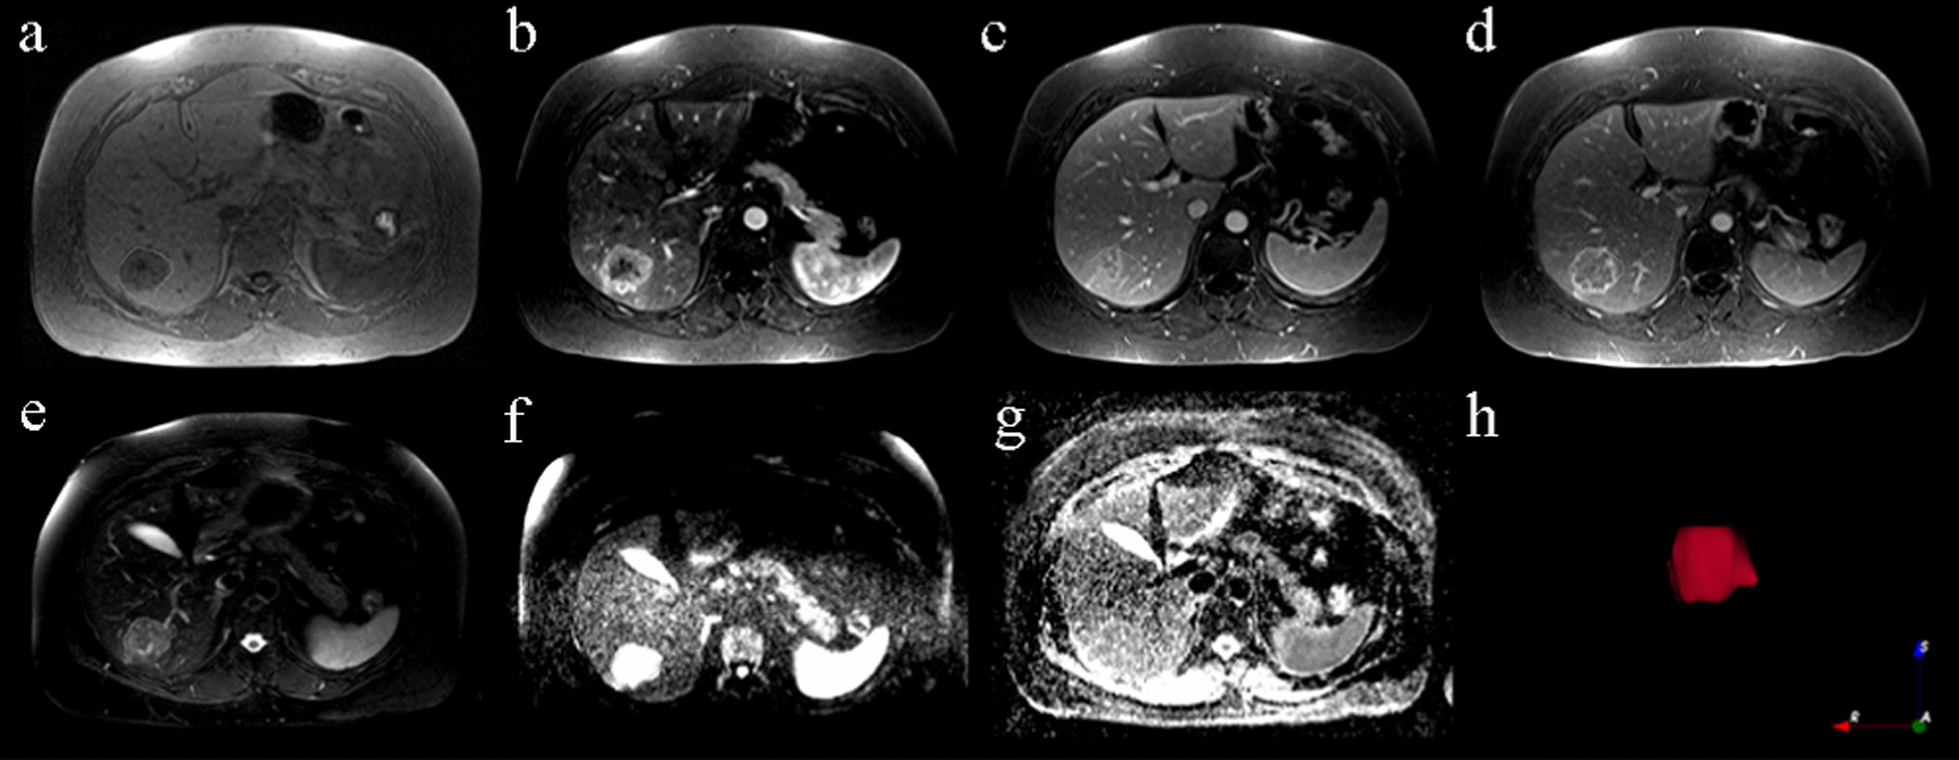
An example of the tumor VOI for different MRI sequences. The VOI were delineated on T2WI, then DWI and ADC sequences shared the same VOI after being registered to T2WI.In addition, T1WI, Portal venous phase and Delay phase sequences shared the same VOI after being registered to Arterial phase. a TIWI, b arterial phase, c portal venous phase, d delay phase, e T2WI, f DWI (b = 800), g ADC, h a three-dimensional rendering of the tumor region
